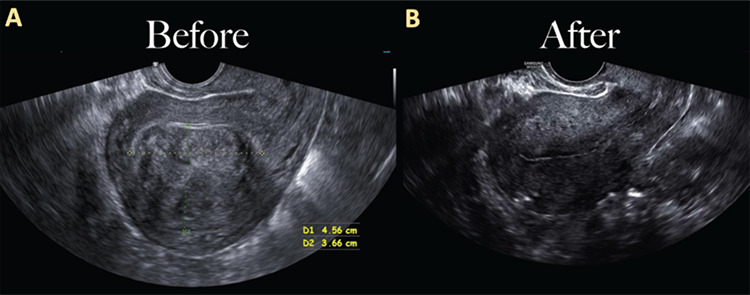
Transvaginal ultrasound of the uterus. Follow up transvaginal ultrasound (B) done 4 months postoperatively showed normalization of uterine anatomy and endometrial contour, compared to the preoperative ultrasound (A)
